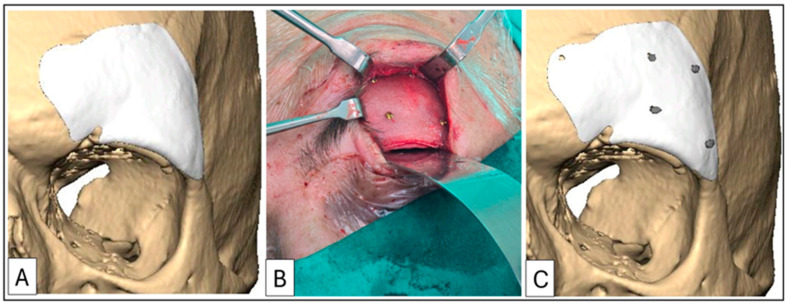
Case 5: A supraorbital rim and frontal bone onlay. (A) Planned implant and positioning. (B) Intraoperative placement and fixation with four 1.5 mm diameter lag screws (All subsequent PMMA implants were fixated using 1.5 mm diameter screws of a designated length, determined during the planning phase based on preoperative CT measurements). (C) Postoperative 3D-CT scan superimposed on planning to confirm correct positioning. Patient 5, a pugilist, required an implant without barium sulphate for enhanced strength. Positioning was confirmed by aligning the lag screws with the planned divots on the implant.
